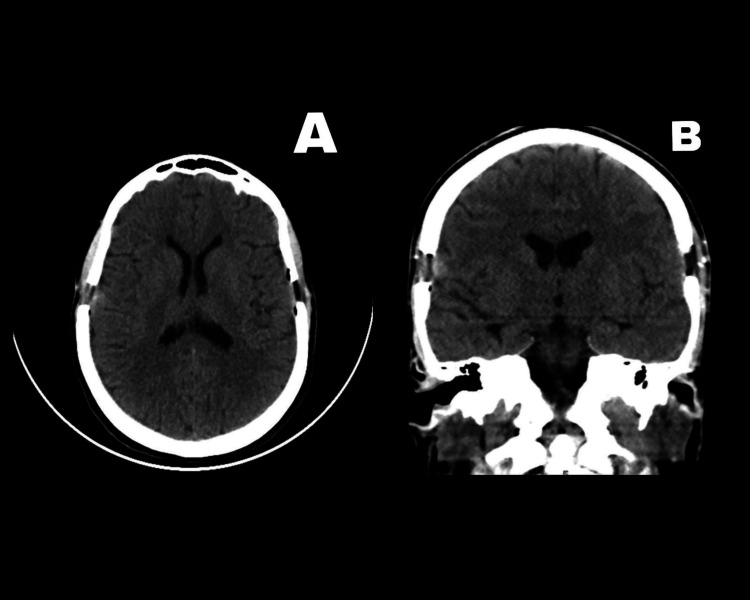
Head CT scan at the follow-up visit showing complete resolution of the hematoma Figure 3A shows the axial view. Figure 3B shows the coronal view CT: computed tomography
